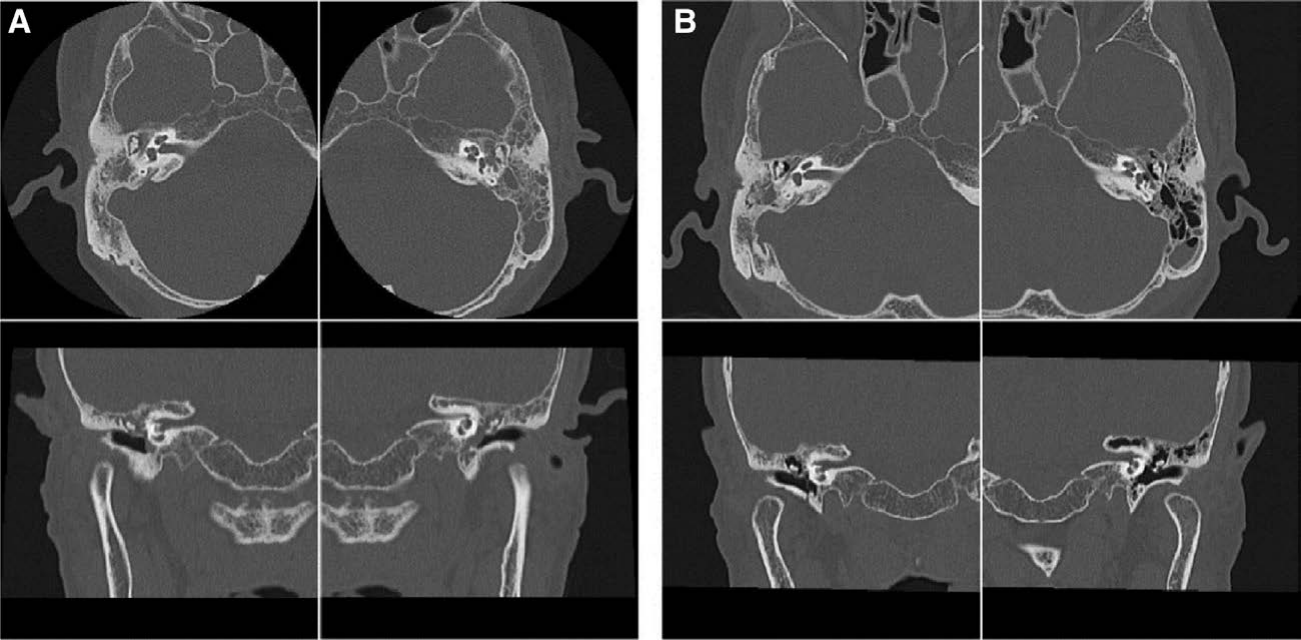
Changes in temporal bone CT image. (a): CT image of the temporal bone before starting treatment. Severe soft tissue opacities are observed bilaterally in the middle ear cavity and mastoid cells. (b): Temporal bone CT image during oral administration of 25 mg PSL/day, about 8 weeks after the start of oral steroid treatment. Bilateral otitis media with effusion improves, and the mastoid cells also puff up. CT = computed tomography, PSL = prednisolone.
